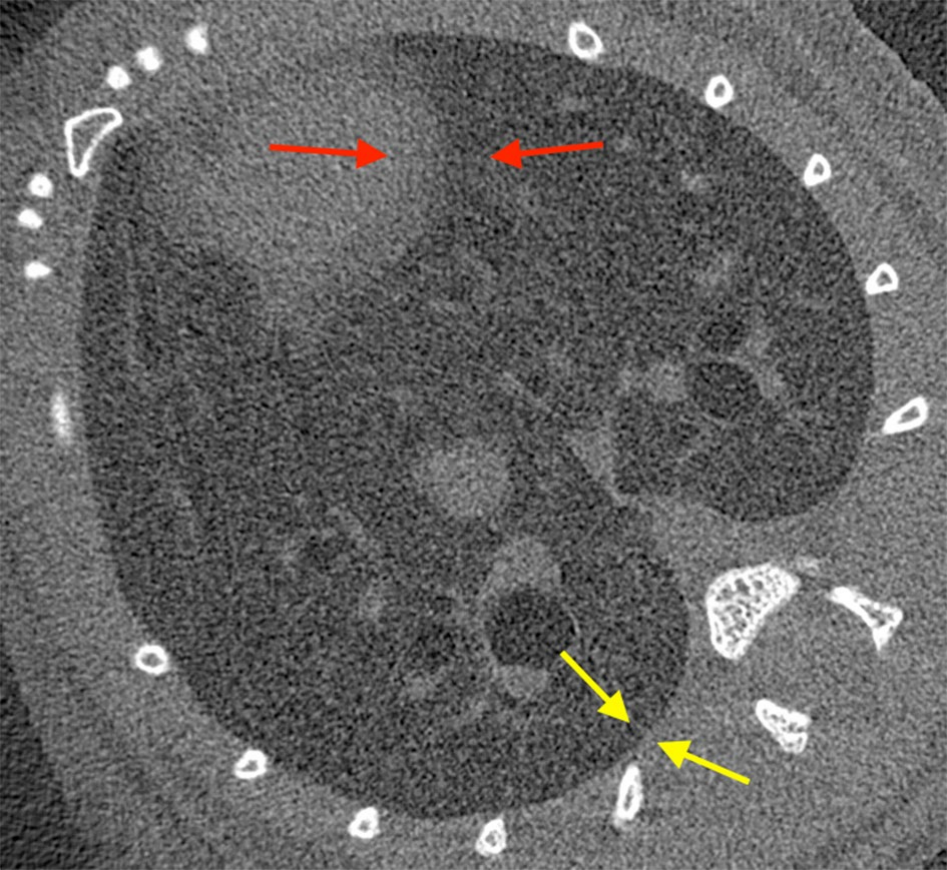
A single CT slice from the first frame of sample M11. The arrows indicate the distinction between a blurred edge (red) between the heart and lung, as a result of heart motion, and a well-defined edge (yellow), more distant from the heart.
